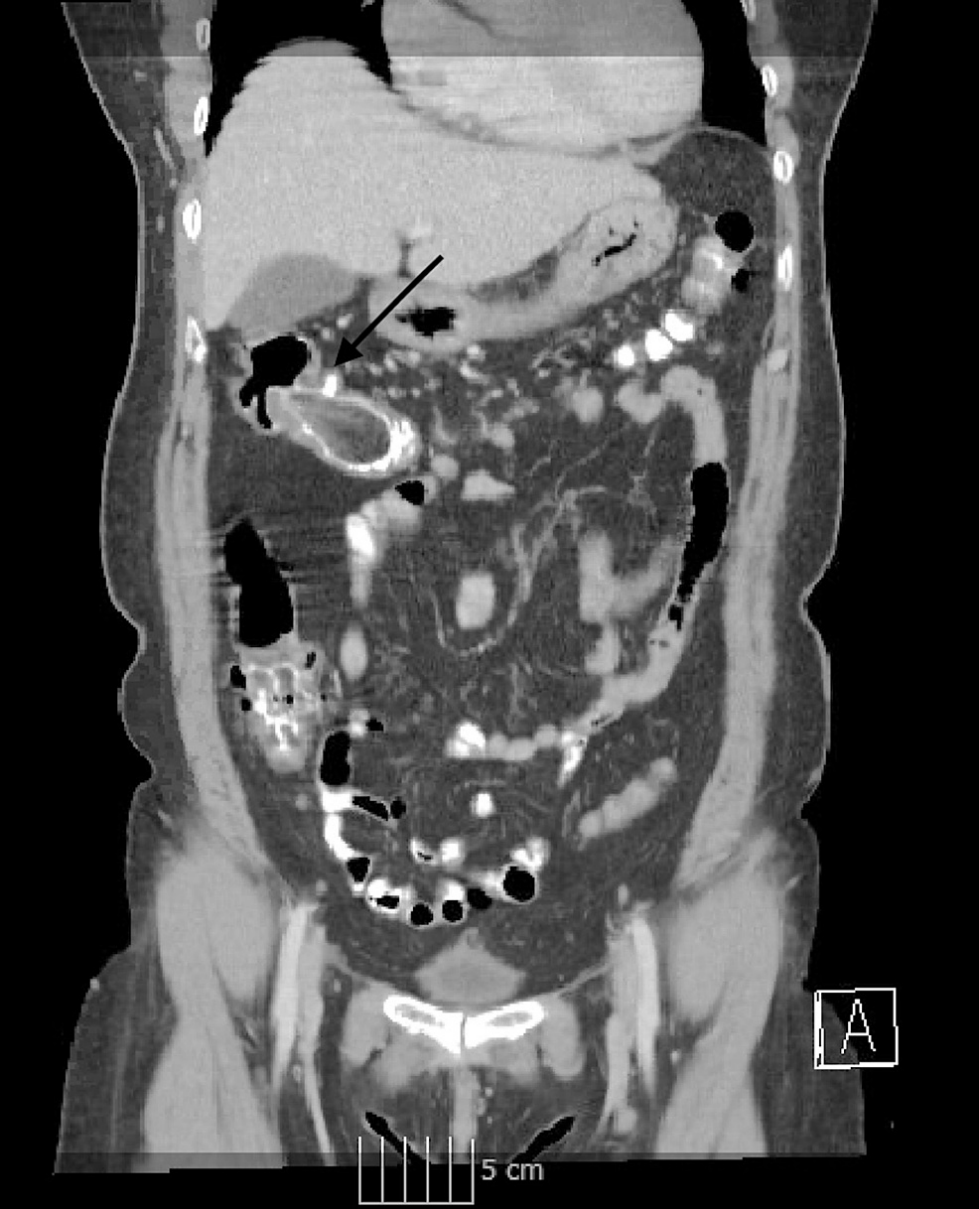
CT demonstrating a giant colonic lipoma with intussusception of the ascending colon (black arrow)
